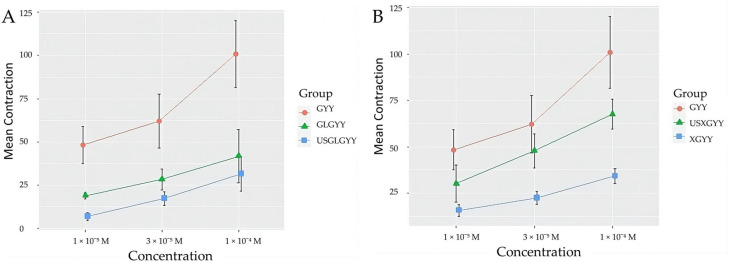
Average contractions induced by GYY 4137 in the absence and the presence of the potassium channel blockers, glibenclamide and XE991. (A)—Average GYY 4137 contraction in the absence (GYY) and the presence of a blocker of ATP-sensitive potassium channels, glibenclamide (GLGYY) and glibenclamide plus low-frequency ultrasound (USGLGYY) compared with each other with two-way (class factors: groups and concentration) analysis of variance (ANOVA): F (12) = [5.25, 7.77], *p* = [0.0231, 0.0069], *n* = 5 per group. (B)—GYY 4137 induced vascular contraction in the absence (GYY) and the presence of a blocker of K_V_7 channels, XE-991 incubation (XGYY) and XE991 plus low-frequency ultrasound (USXGYY), compared with each other with two-way (class factors: groups and concentration) analysis of variance (ANOVA): F (12) = [3.73, 21.93], *p* = [0.0550, <0.0001], *n* = 5 per group. The graphs represent the vascular response to increasing concentrations of GYY4137. Data are represented as mean ± S.E.M.
